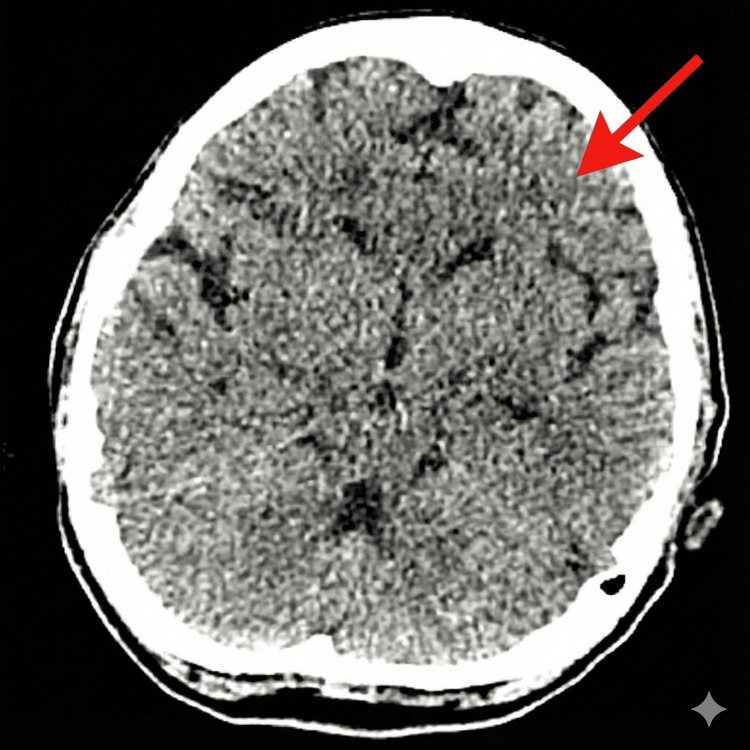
A CT of the brain demonstrating features of hypodensities in the frontal lobe.
